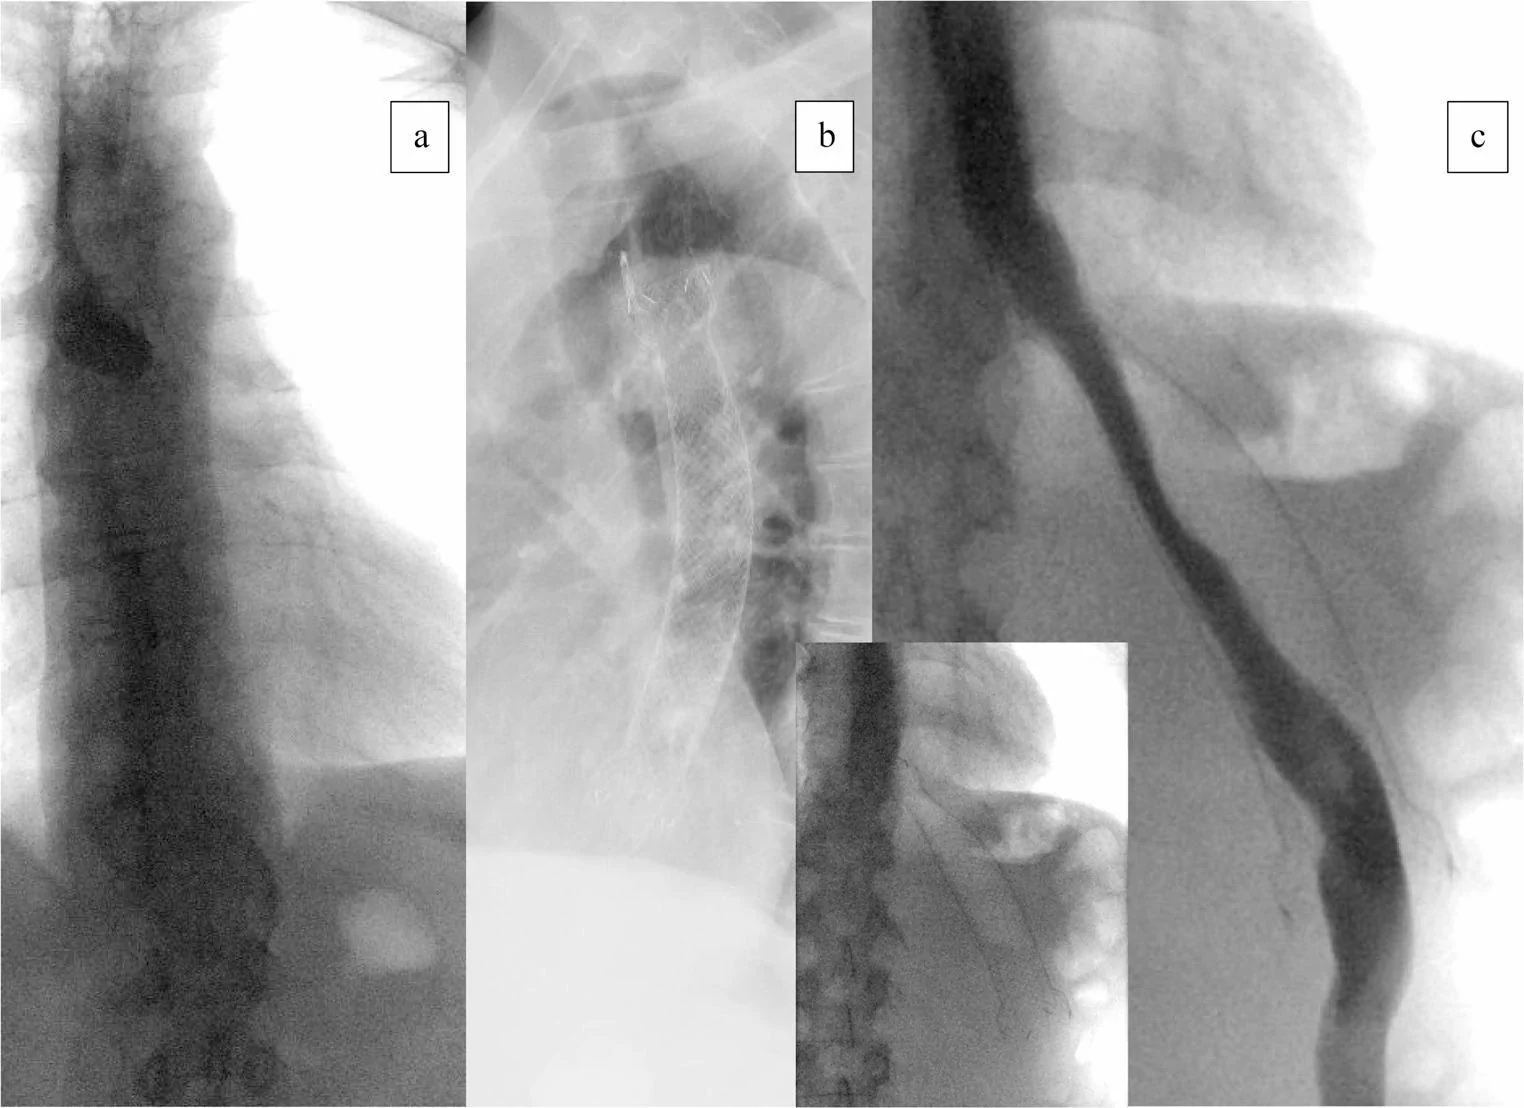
Contrast agent clearance before (a) and after (b,c) SEMS-ART insertion via x-ray
